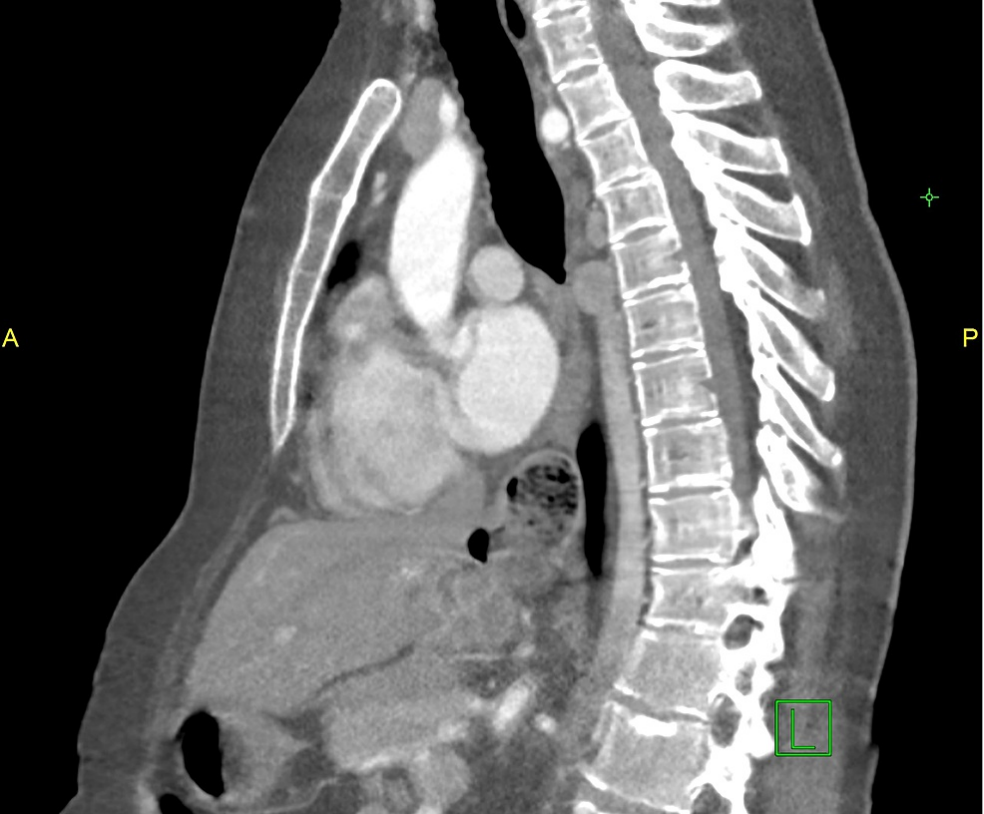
Lateral abdominal CT scan image showing the azygous continuation of inferior vena cava CT: computed tomography
